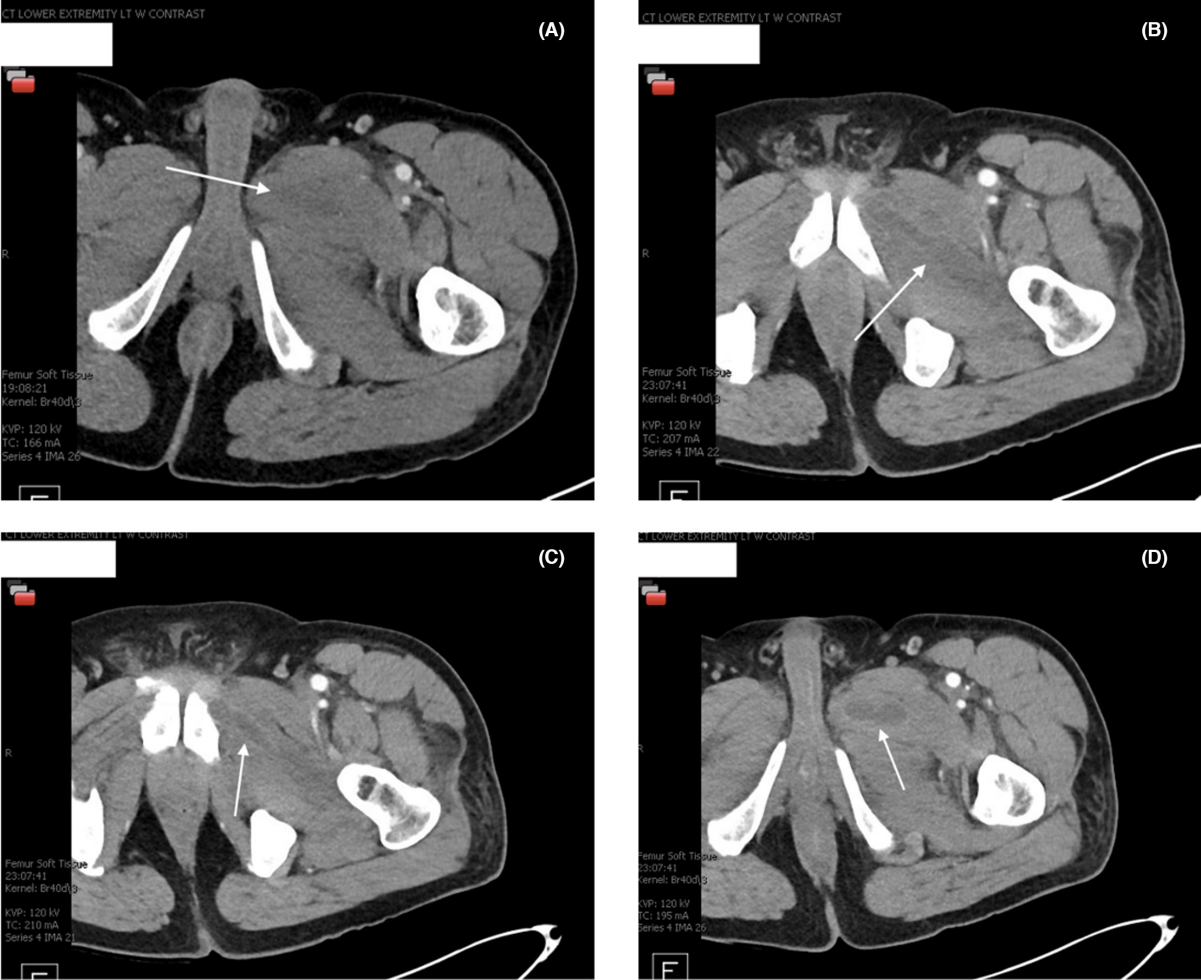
(A, B) Computed tomography (CT) with contrast of lower extremity showing evidence of myositis. (C, D) Repeat CT with contrast of lower extremity showing abscesses.
